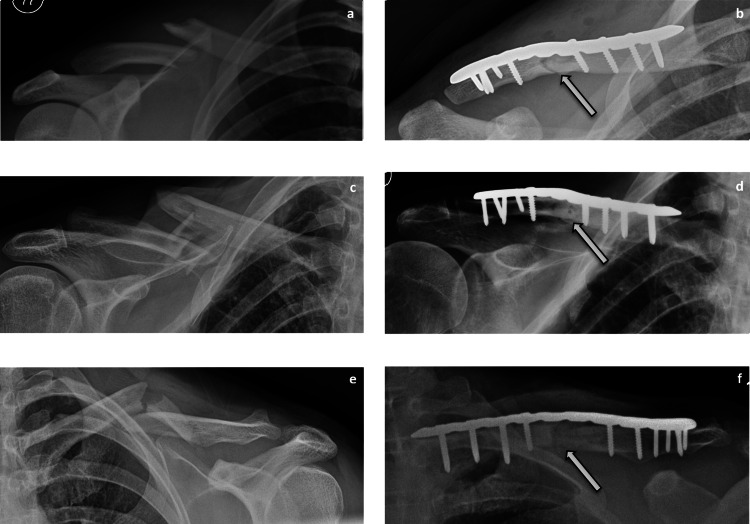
Pre- and post-clavicle fixation radiographs demonstrating union, as denoted by arrows.
